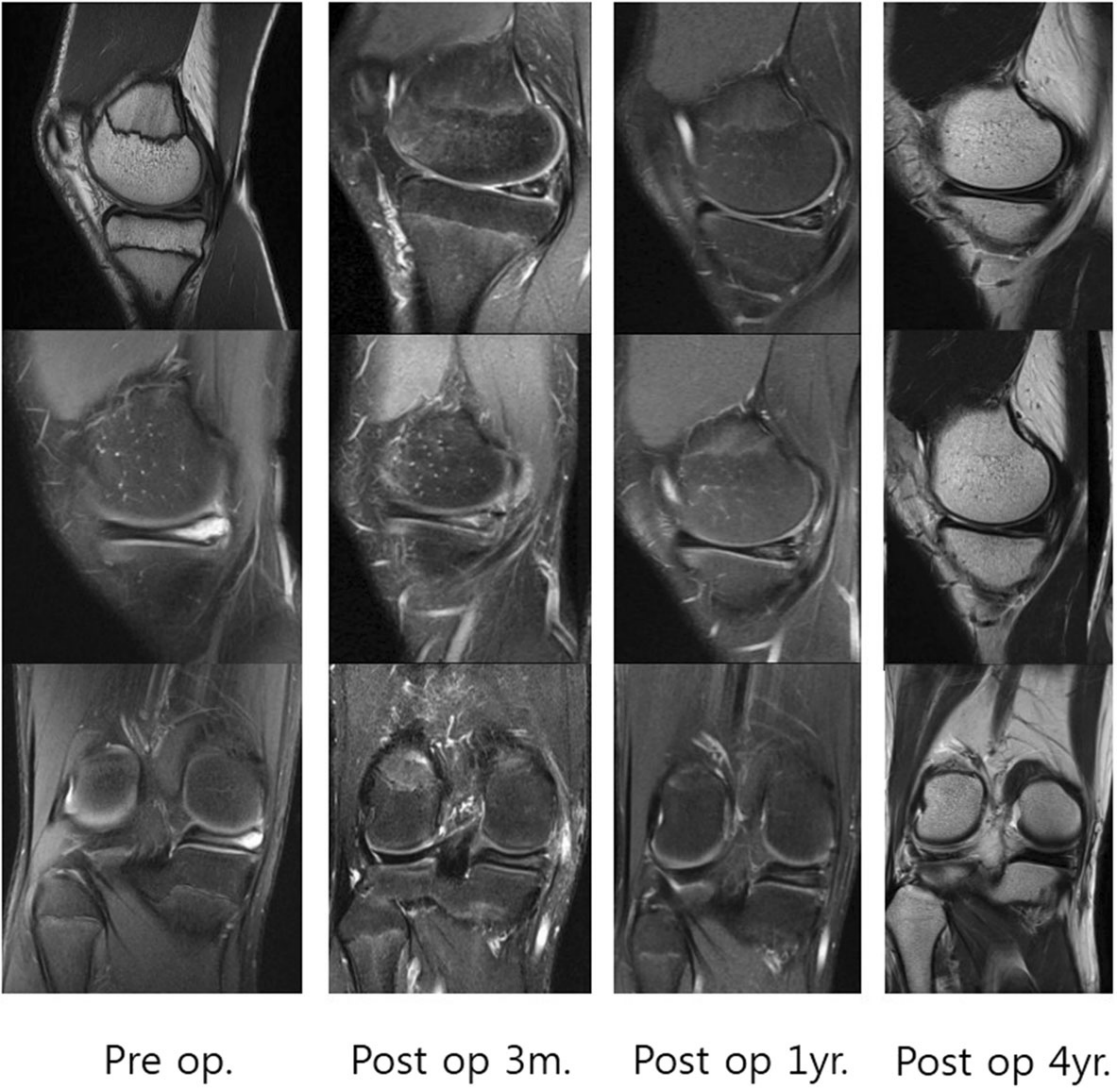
Pre op. Magnetic resonance images showing a huge intrameniscal cyst located in the posteromedial corner of the medial meniscus accompanied by a very thin meniscal parenchymal layer between the cyst and the upper and lower surfaces of the meniscus. Vertical tear of the upper meniscal surface in the red-white zone of the posteromedial corner of the medial meniscus. Post op. Follow-up magnetic resonance images taken about 3 months, 1 year, 4 years postoperatively showing that the high signal intensity where the meniscal cyst was slowly disappeared over time. Four year postoperatively showing a normalized meniscus
